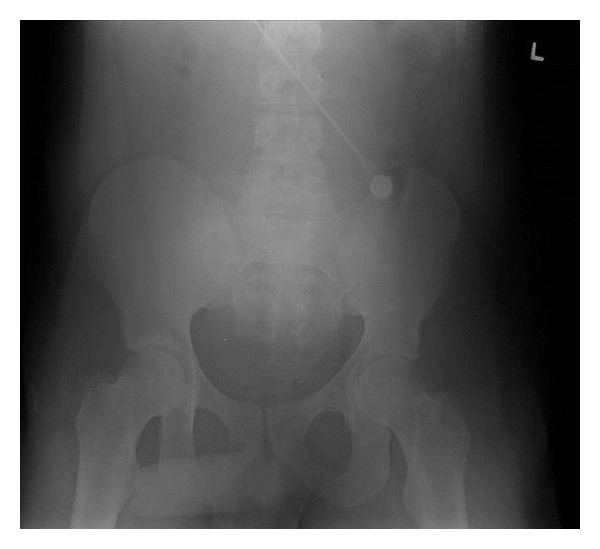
Pelvic X-ray did not reveal any fracture or radiopaque foreign body.
